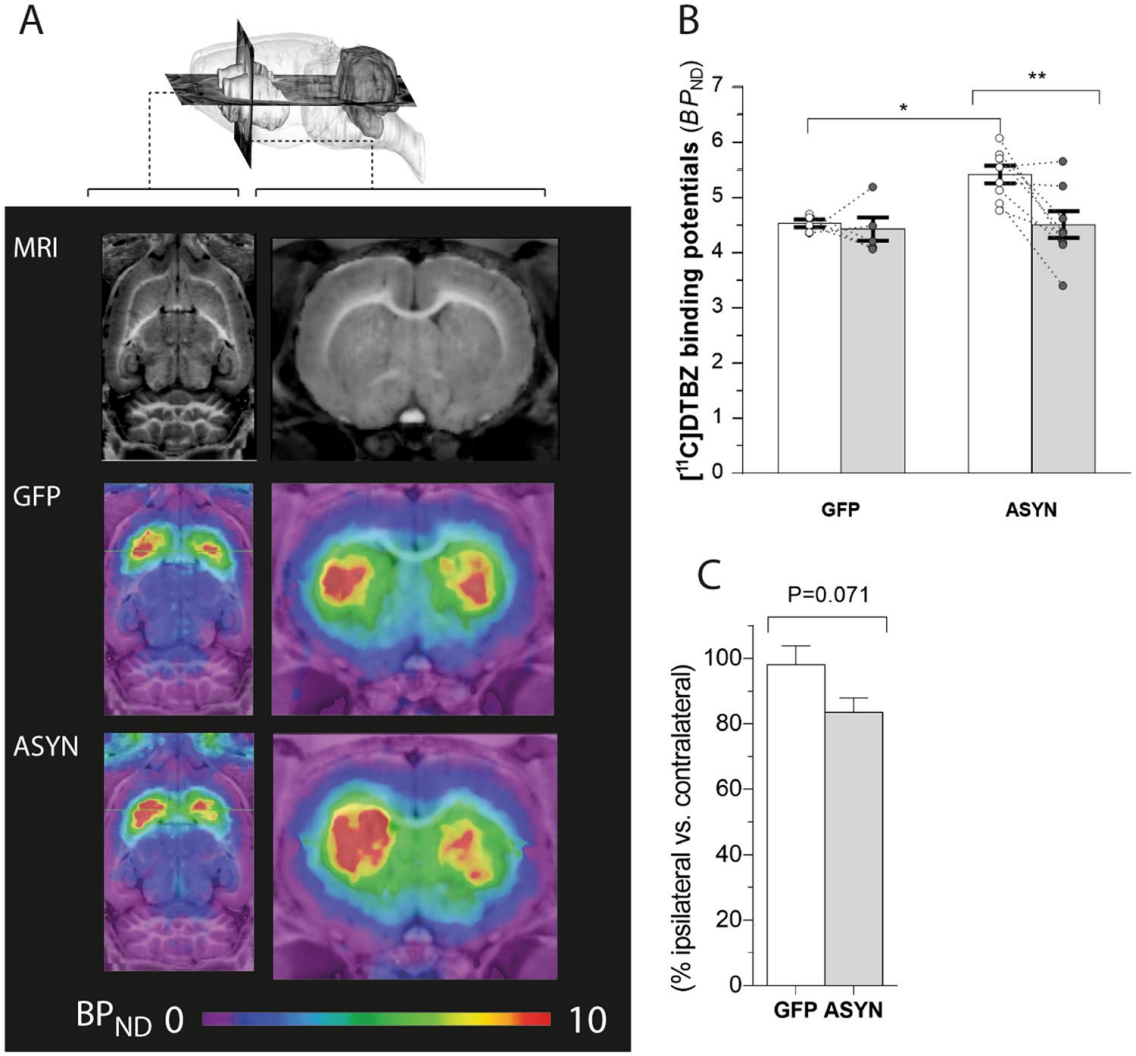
In vivo* VMAT2 expression determined by [^11^C]DTBZ PET. Representative axial (A, left) and coronal (A, right) sections of MRI atlas (top row) and parametric maps of *in vivo* [^11^C]DTBZ binding potentials (*BP*
